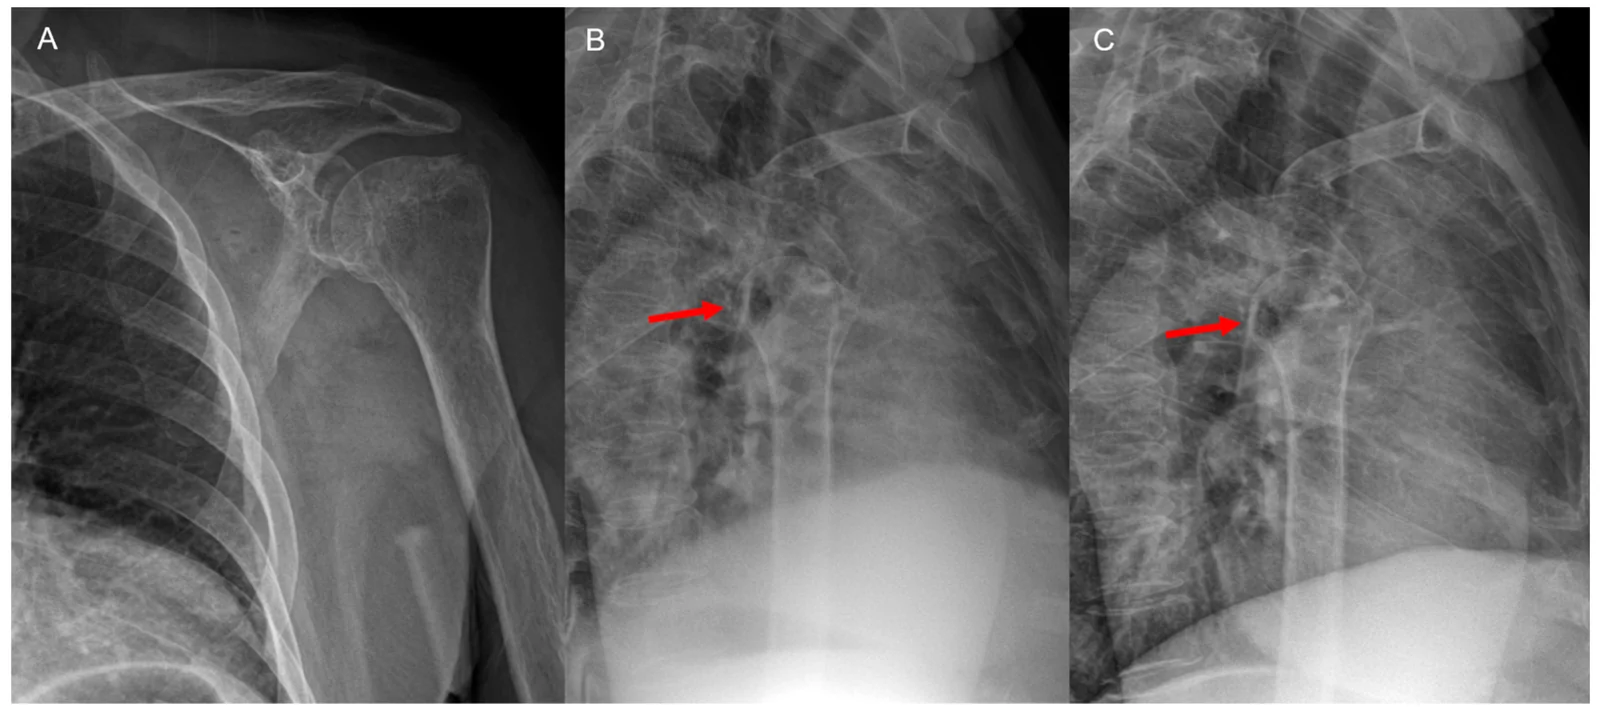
Shoulder X-ray radiographs of a 65-year-old male patient with degenerative shoulder disease. (A) Anteroposterior shoulder X-ray image showed focal cortical hyperostosis. The anatomical marginal delineation of the humerus was significantly inferior, as shown on routine free-breathing (B), when compared with a deep-inspiration breath-hold transthoracic lateral shoulder radiograph (C) (arrows).
